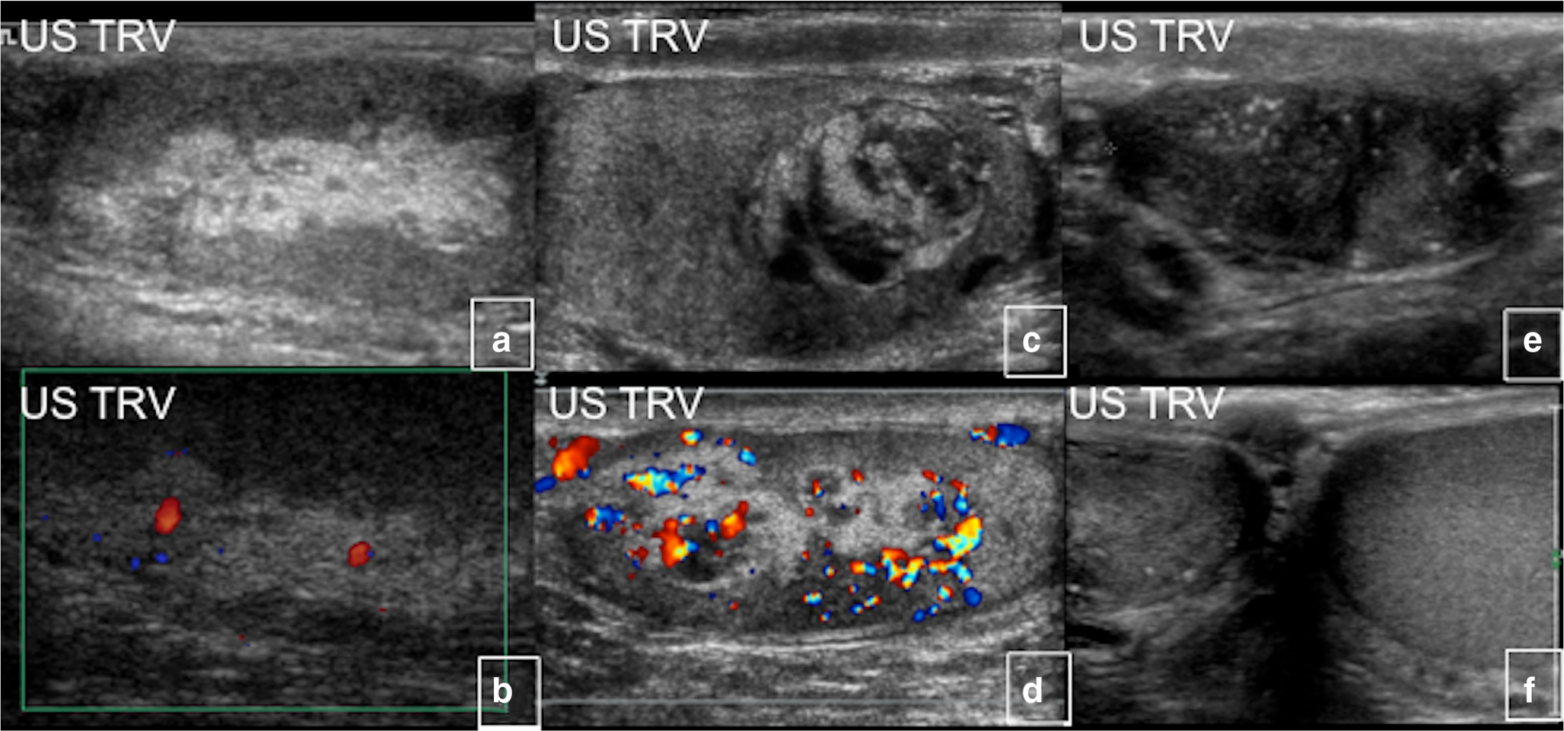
Testicular abnormalities in McCune-Albright syndrome in three different patients. a, b Ultrasound (US) of the testicles shows extensive echogenic material secondary to Leydig cell hyperplasia. c, d The heterogeneous appearance of the testicle with more focal, well-circumscribed areas of abnormal echogenicity. These imaging findings correlate with Leydig cell hyperplasia on pathology. e, f The right testicle appears atrophic and demonstrates inhomogeneous echotexure and multiple punctate calcifications
